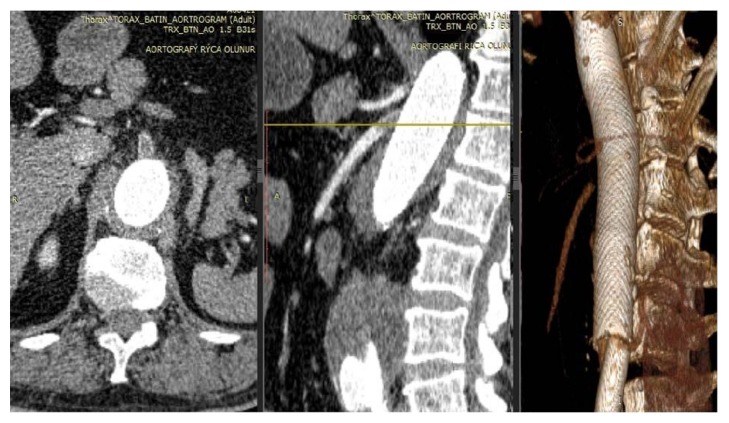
Images of CT angiography which showed the occlusion of SMA and the Coeliac due to thrombus.
